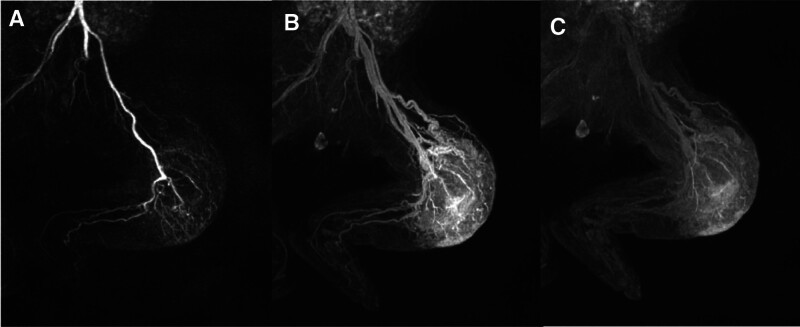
(A) MRI enhancement in the arterial phase shows that the tumor is supplied by a branch of the femoral artery. (B/C) MRI enhancement in the venous and delayed phases show tumor invasion of the muscular layer and reflux through the femoral vein.
